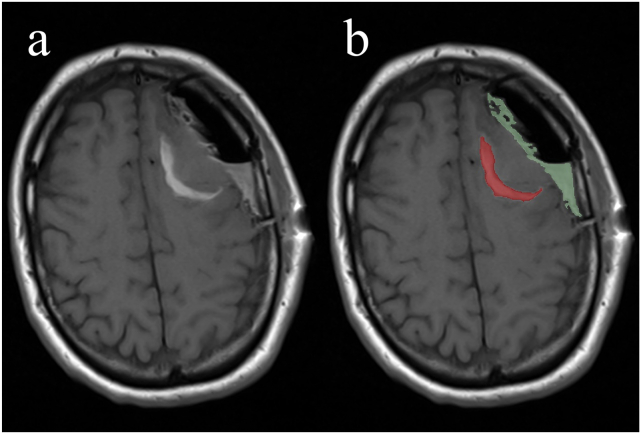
T1-weighted non-enhanced MRI scan exemplifying segmentation of postoperative intradural and extradural blood; a: unedited MRI scan; b: MRI scan after segmentation of blood.
